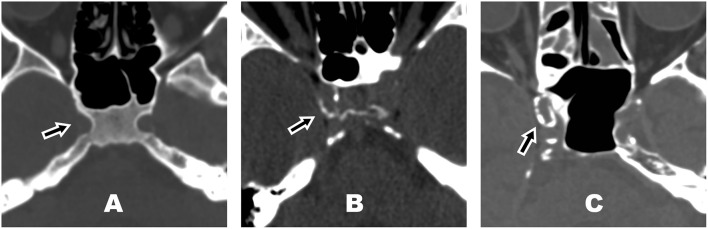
Examples of different degrees of intracranial carotid artery calcification in the right carotid artery (arrow) on unenhanced bone-window computed tomography (CT) image. (A) Intracranial carotid artery calcification (ICAC) score = 0 (no calcification), (B) ICAC score = 2 (mild, 10–50% of the vessel circumference constituted of calcification), (C) ICAC score = 4 (severe, >90% of the vessel circumference constituted of calcification).
